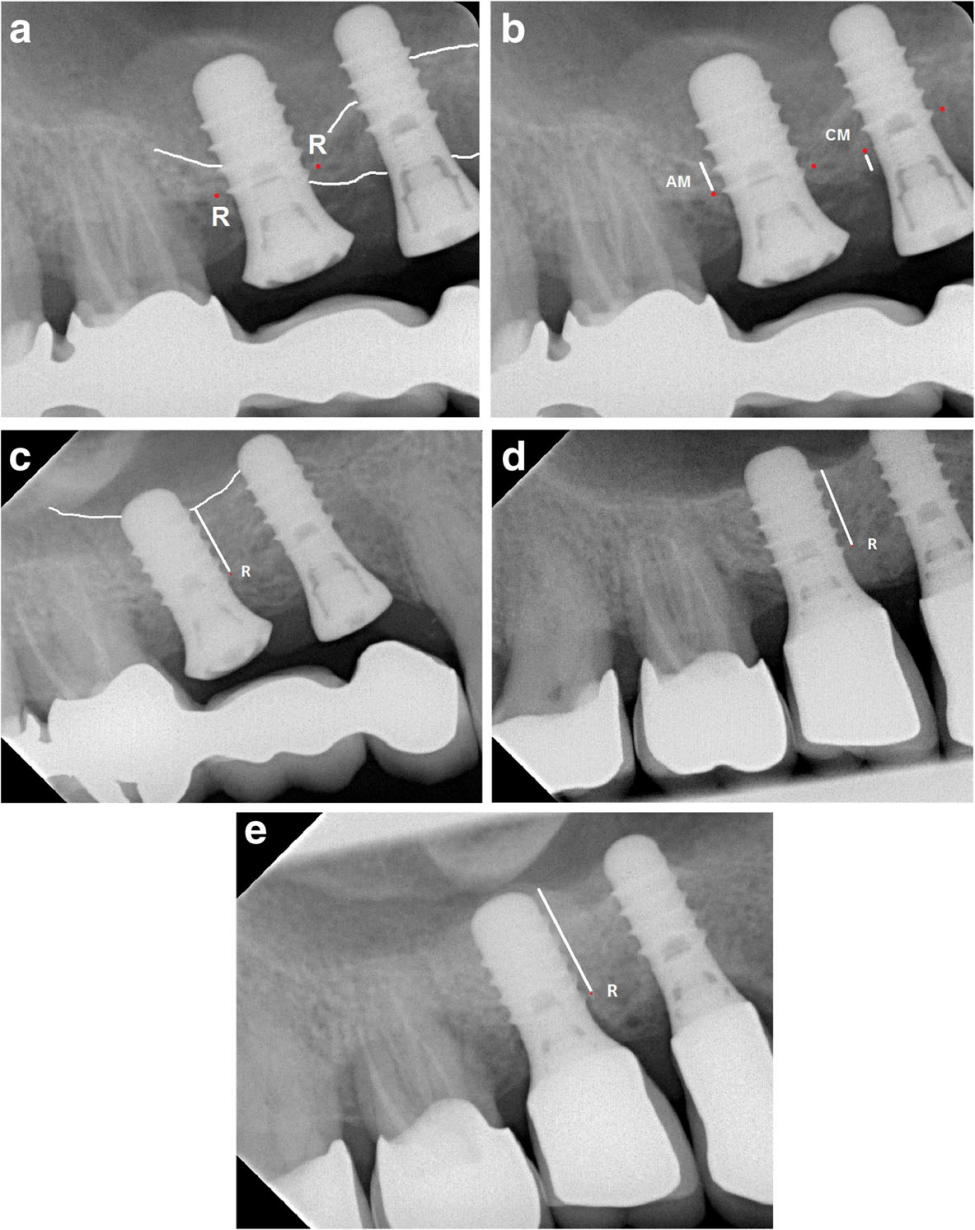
Radiographs showing measurements of bone heights (bone edges, marginal and sinus floor, with white lines) using the first coronal thread as reference point R (red dots). a post-operative; b after 6 months; c after 2 years; d after 6 years; e after 10 years. Legend: R = reference point (first coronal thread of the implant); AM = apical measurement (distance between R and the sinus floor); CM = coronal measurement (distance between R and the marginal bone edge). Note: For reason of clarity R, AM and CM are sketched at two implants. The complete measurements (mesial and distal) were performed for all implants. In the radiograph c) it can be seen that the implants are still not loaded. In some cases incorporation of final prostheses was prolonged due to participants’ causes, e.g. financial restrictions
